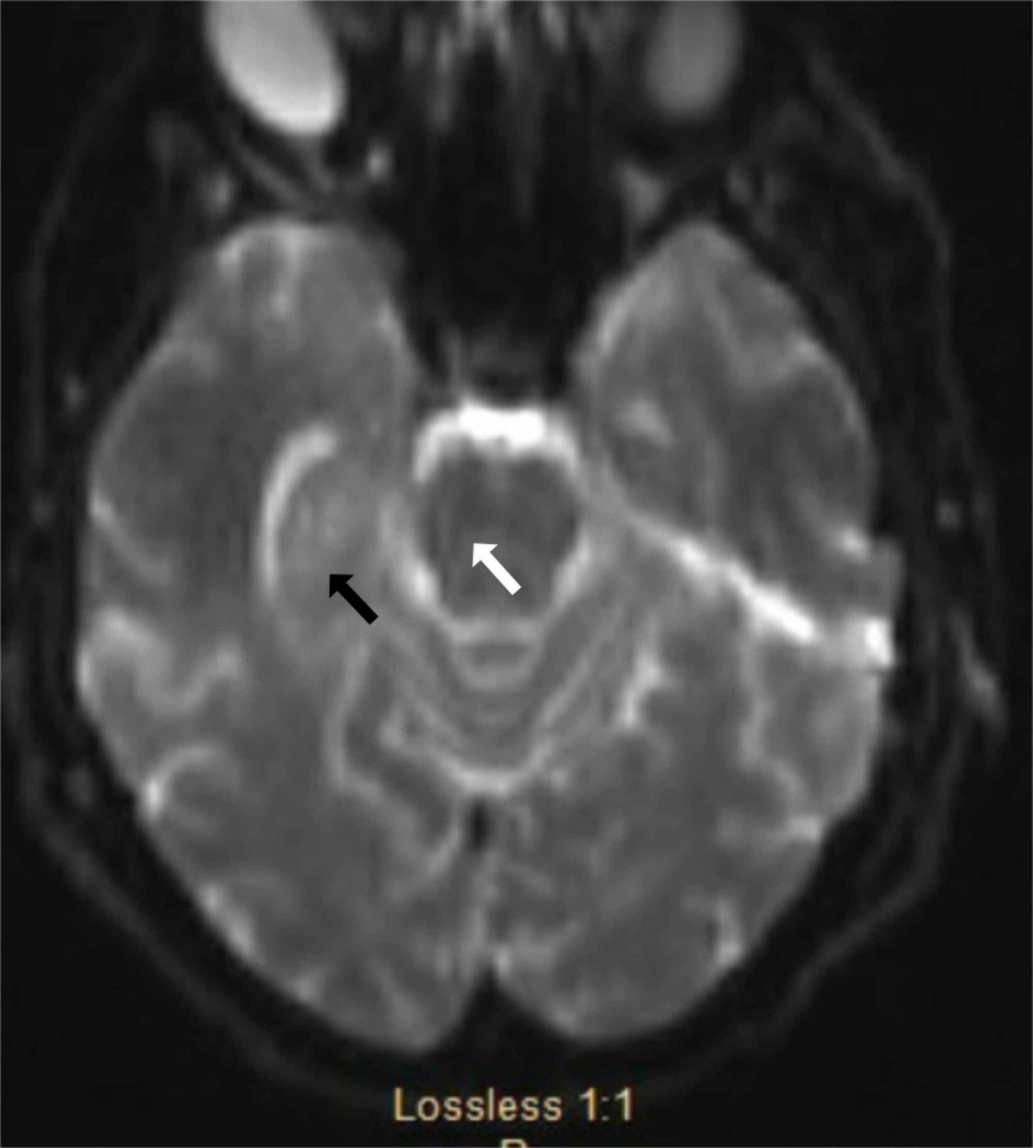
MRI of the brain demonstrates small vessel disease (black arrow) and a right parietal and pontine cavernoma (white arrow).
